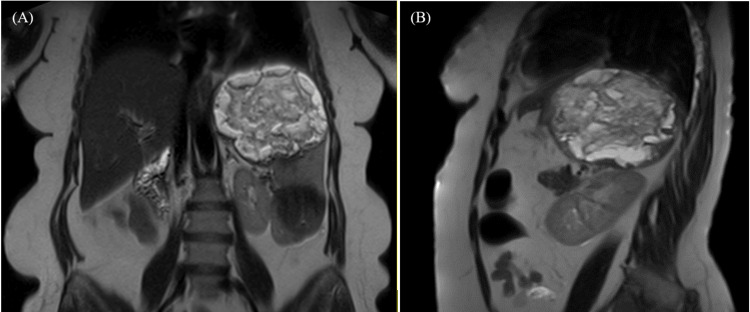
Preoperative abdominal MRI imaging. Coronal (A) and sagittal (B) MRI images reveal marked splenomegaly with mass-like formation.
